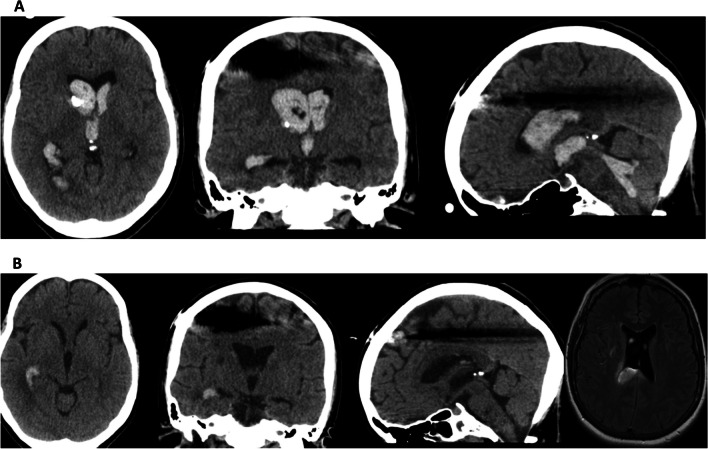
A Patient with intracranial haemorrhage and intraventricular haemorrhage before active cerebrospinal fluid exchange. The removal of blood was achieved in 47 h with a total of 2.4 mg tissue plasminogen activator in irrigation fluid. B MRI shows oedema only in part of brain parenchyma where removal of blood was not sufficient
